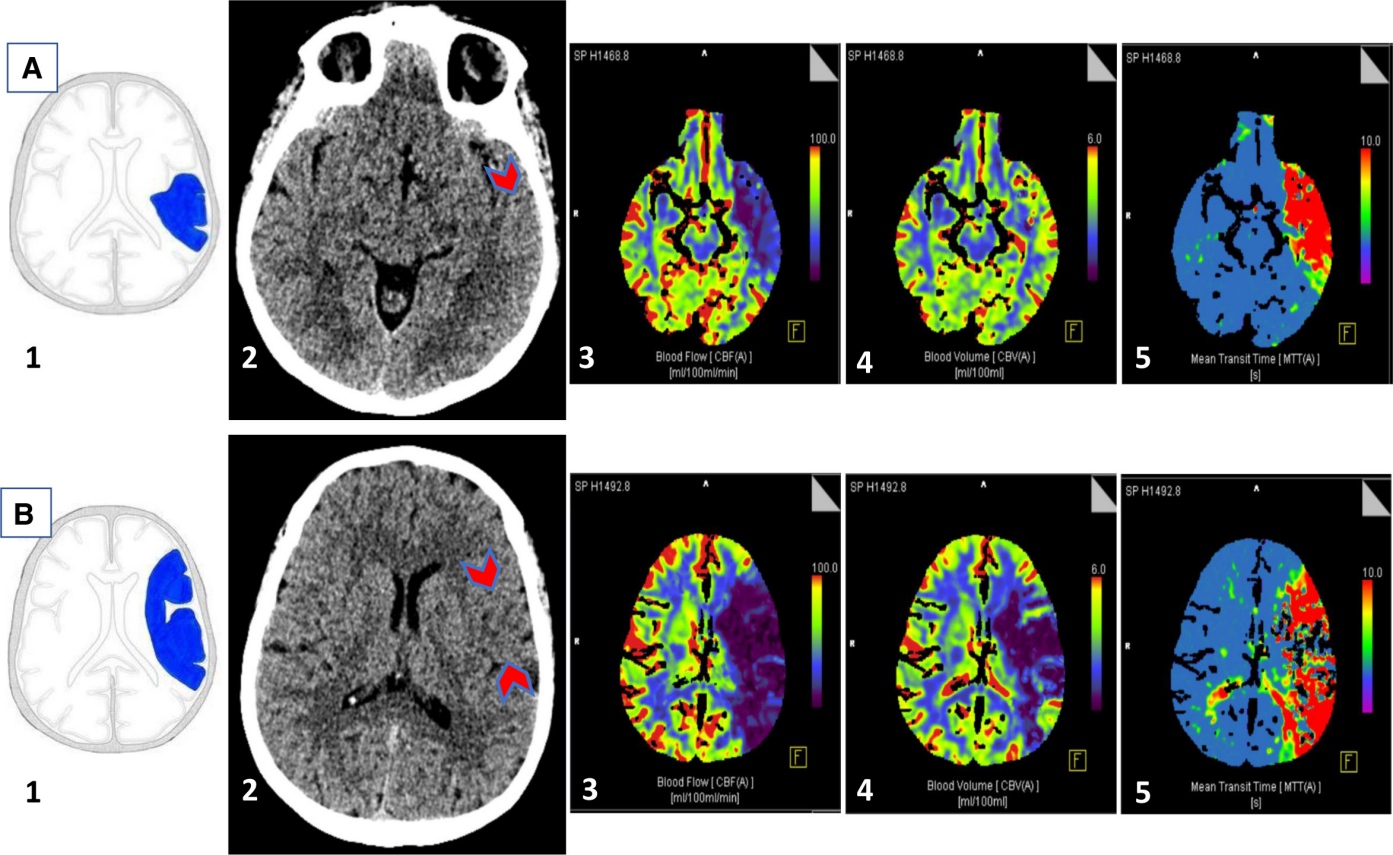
Example computed tomography (CT) scan assessment of 2 brain regions from the IST-3 trial (Third International Stroke Trial) template for the same patient. A, (1) Posterior half of peripheral middle cerebral artery (MCA) territory, (2) isolated focal swelling on nonenhanced CT (NECT), (3) decreased cerebral blood flow (CBF), (4) normal cerebral blood volume (CBV), and (5) increased mean transit time (MTT). B, (1) most of peripheral MCA territory + lateral part of basal ganglia, (2) subtle parenchymal hypoattenuation on NECT, (3) decreased CBF, (4) decreased CBV, (5) increased MTT.
